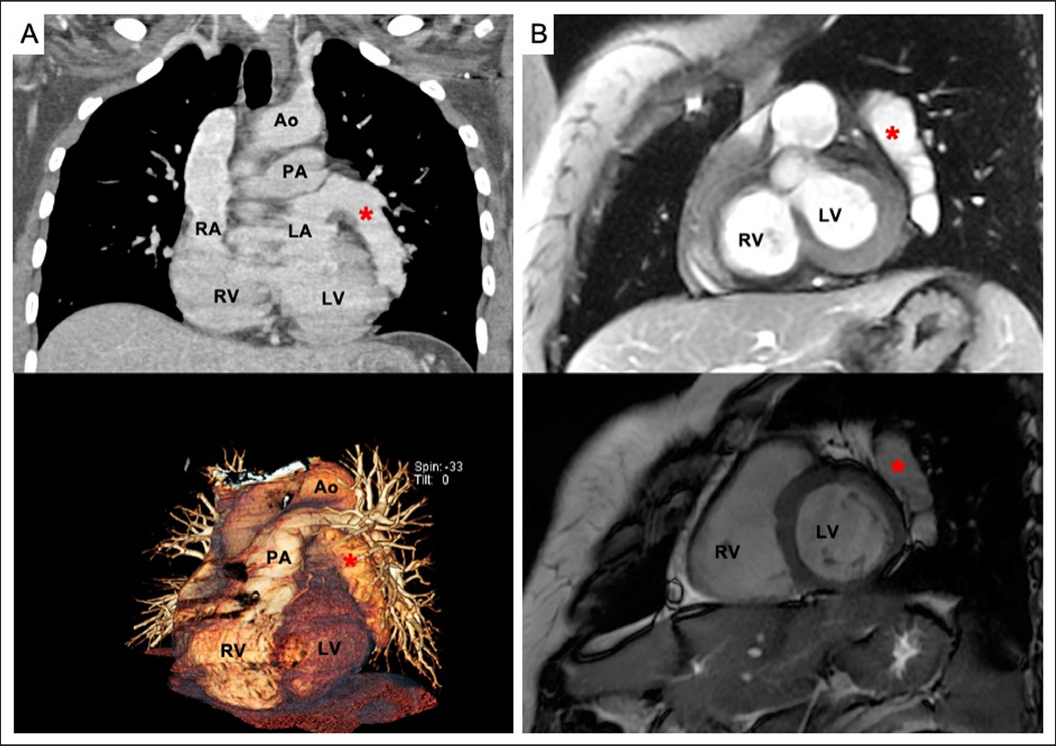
(A) Computed tomography angiography showed a ground-glass opacity and a giant left atrial appendage (red asterisk). (B) Cardiac magnetic resonance confirmed the huge left atrial appendage (red asterisk). Ao: aorta: PA: pulmonary artery; RA: right atrium; RV: right ventricle; LA: left atrium; LV: left ventricle.
